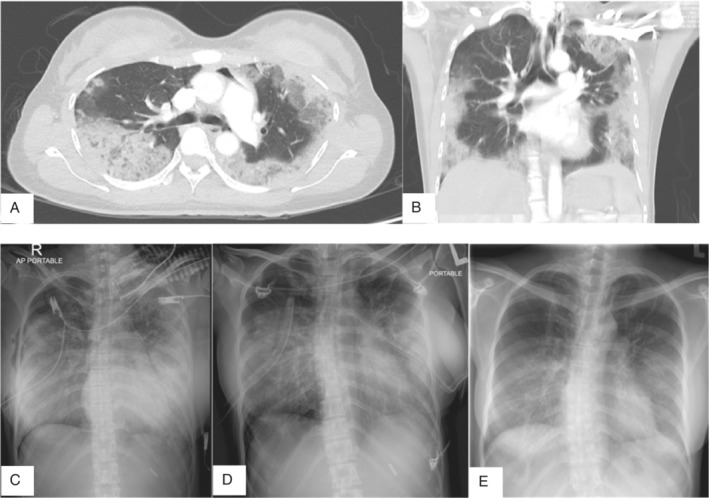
A computed tomography scan of the chest (A and B) shows bilateral, diffusely distributed ground‐glass opacities with superimposed dependent areas of consolidation. Chest X‐ray (C) shows diffuse alveolar opacities of both lung fields. (D) Chest X‐ray with bilateral alveolar opacities and pneumothorax with chest tube in‐situ. (E) Chest X‐ray improvement of bilateral alveolar opacities at 6 weeks of outpatient follow‐up.
